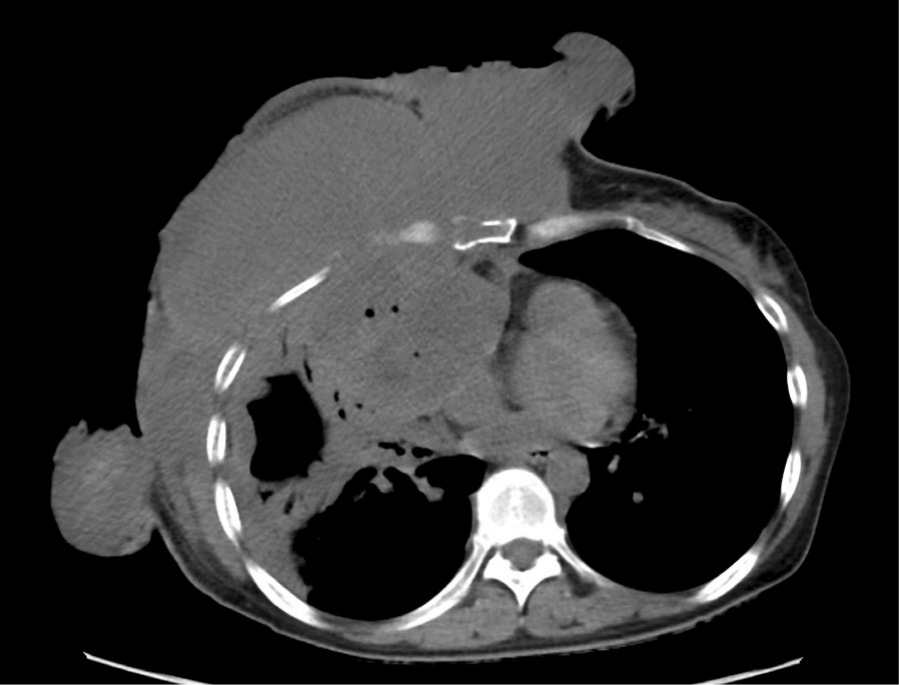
Computed tomography showed a rapid increase in right chest wall tumors and pleural dissemination
